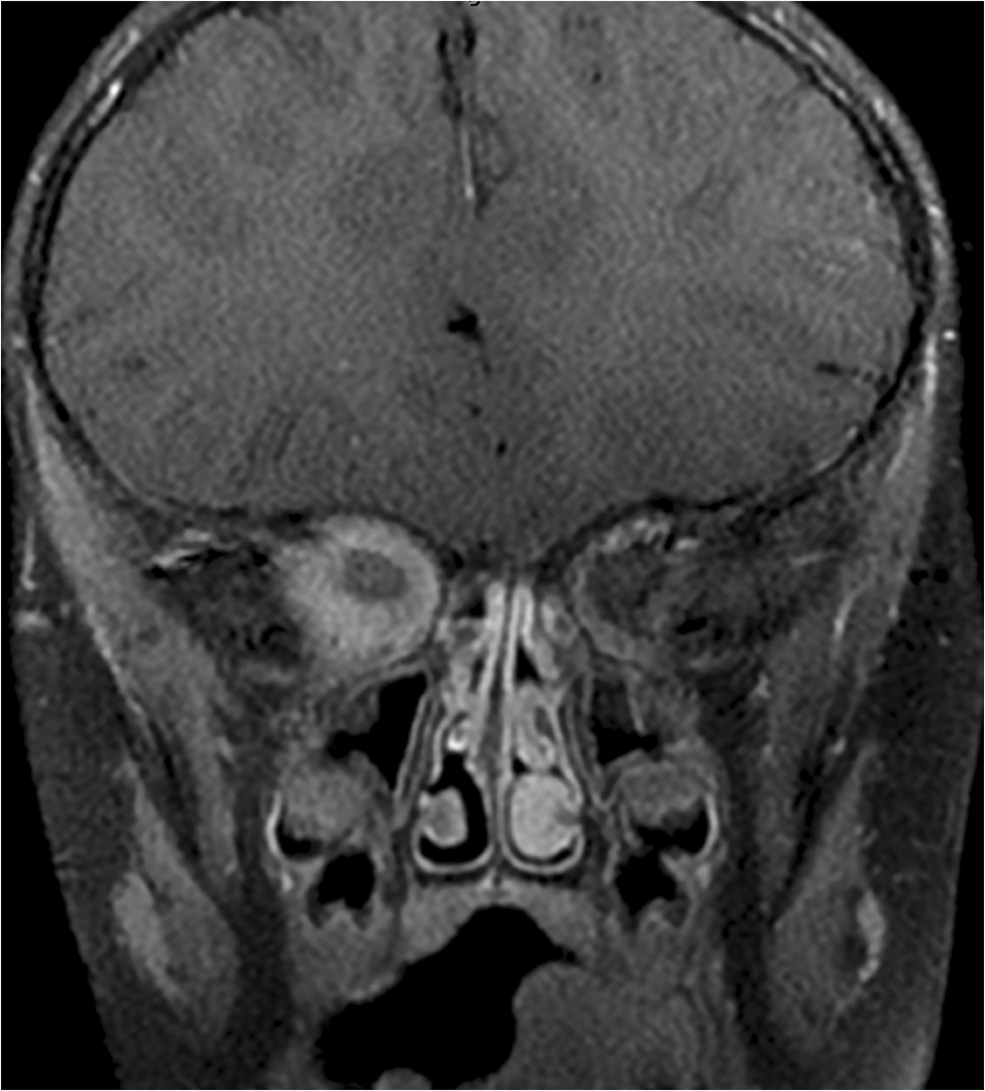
Optic nerve glioma mimicking optic nerve sheath meningioma. The postcontrast coronal T1-weighted image through the orbit shows the tumor around the central hypointense, minimally enlarged right optic nerve. There is no appreciable enhancement of the optic nerve proper. Minimal nerve enlargement may be the only difference between optic nerve glioma and optic nerve sheath meningioma
